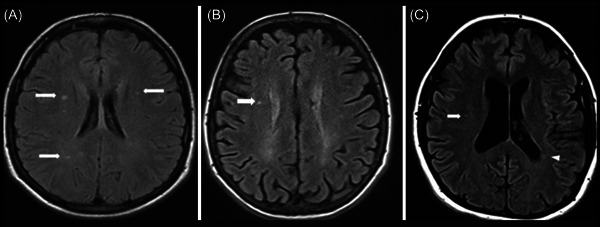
Three patients diagnosed with primary‐progressive multiple sclerosis had focal white matter changes. (A) Axial FLAIR images with a punctate lesion in the right deep white matter (white arrow); (B) axial FLAIR images with only a few small, focal white matter lesions located in the deep white matter of both the left and right brain hemispheres (white arrows); and (C) axial FLAIR images with small, focal lesions located in the right brain hemisphere's deep white matter (white arrow) and periventricular white matter, Figure from Siger.^42^
